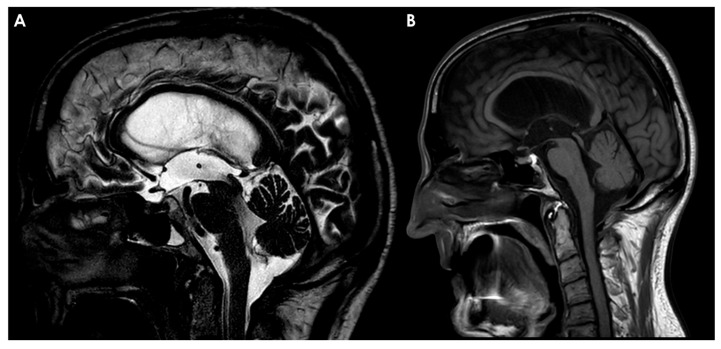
Sagittal MRI (1.5 T) in a 70-year-old patient with hydrocephalus, showing in T2 Drive (A) and in T1W (B) sequences the sellar segment of the LM.
